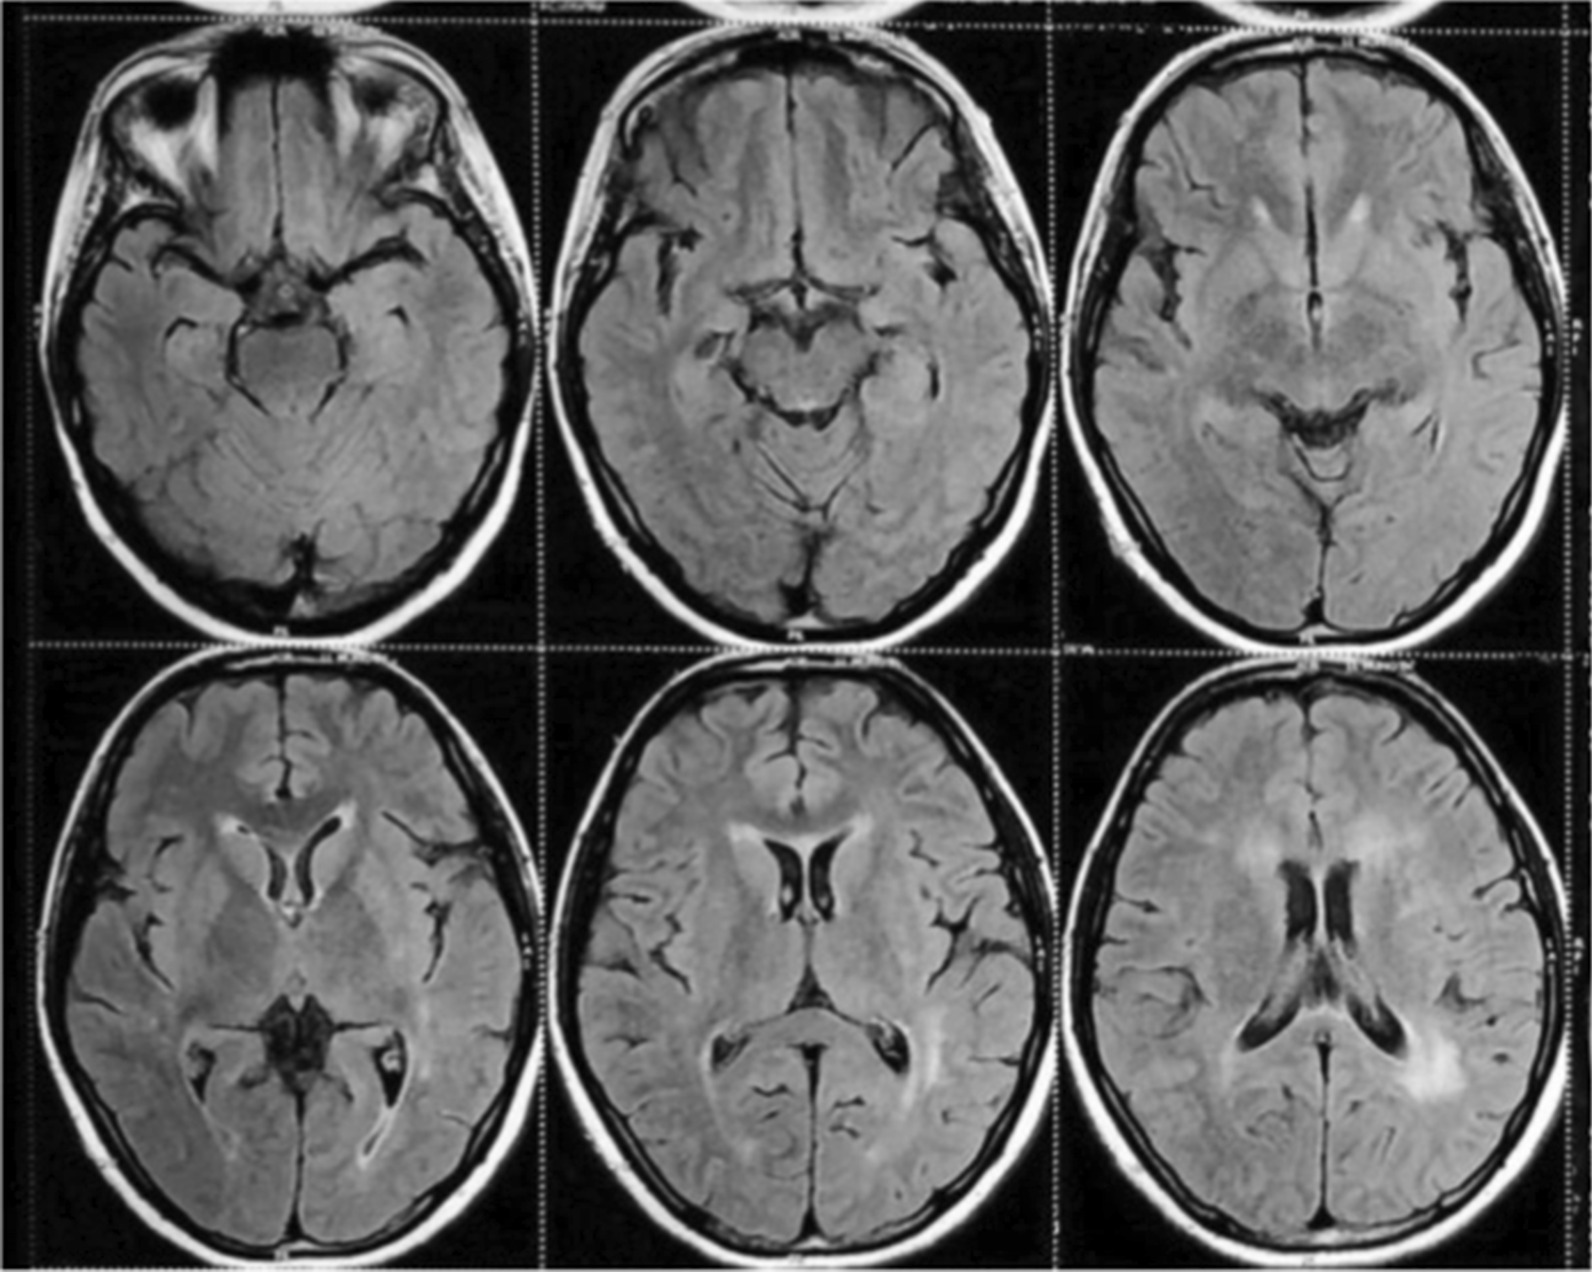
Follow-up MRI brain showing. Resolution of oedema
